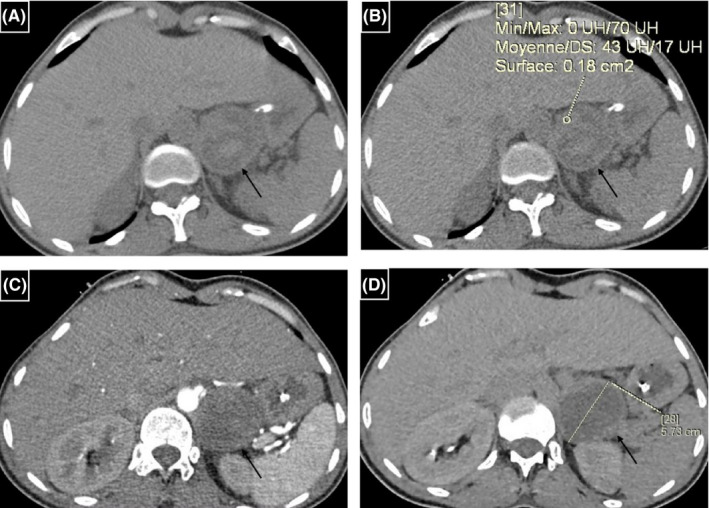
Adrenal mass on the left side (arrows): (A, B) Unenhanced CT scan contrast‐enhanced CT scans in the axial plane, (B) contrast‐enhanced CT scan (arterial phase) in the axial plane, and (D) contrast‐enhanced CT scan (delayed phase) in the axial plane. The lesion has a regular spherical shape, smooth margin, and central necrosis. Unenhanced images have shown areas of high attenuation (43HU) suggesting bleeding changes
